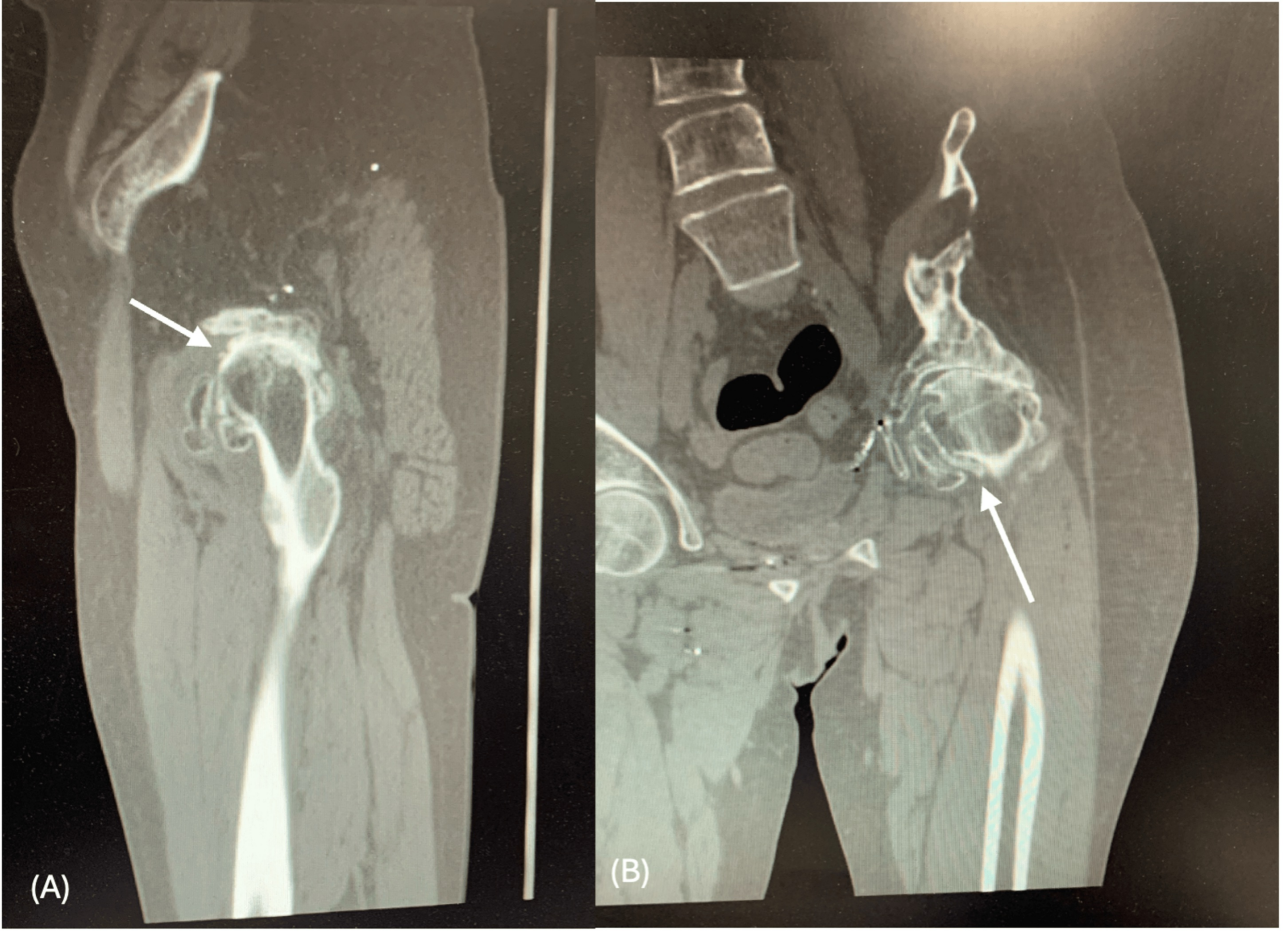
Preoperative computed tomography (MAKO protocol) of the pelvis and left hip. (A) Lateral view demonstrating advanced degenerative changes with osteophyte formation and anterior heterotopic ossification. (B) Anteroposterior view showing chronic left iliac wing fracture deformity with retained metallic fragments and associated pelvic tilt.
